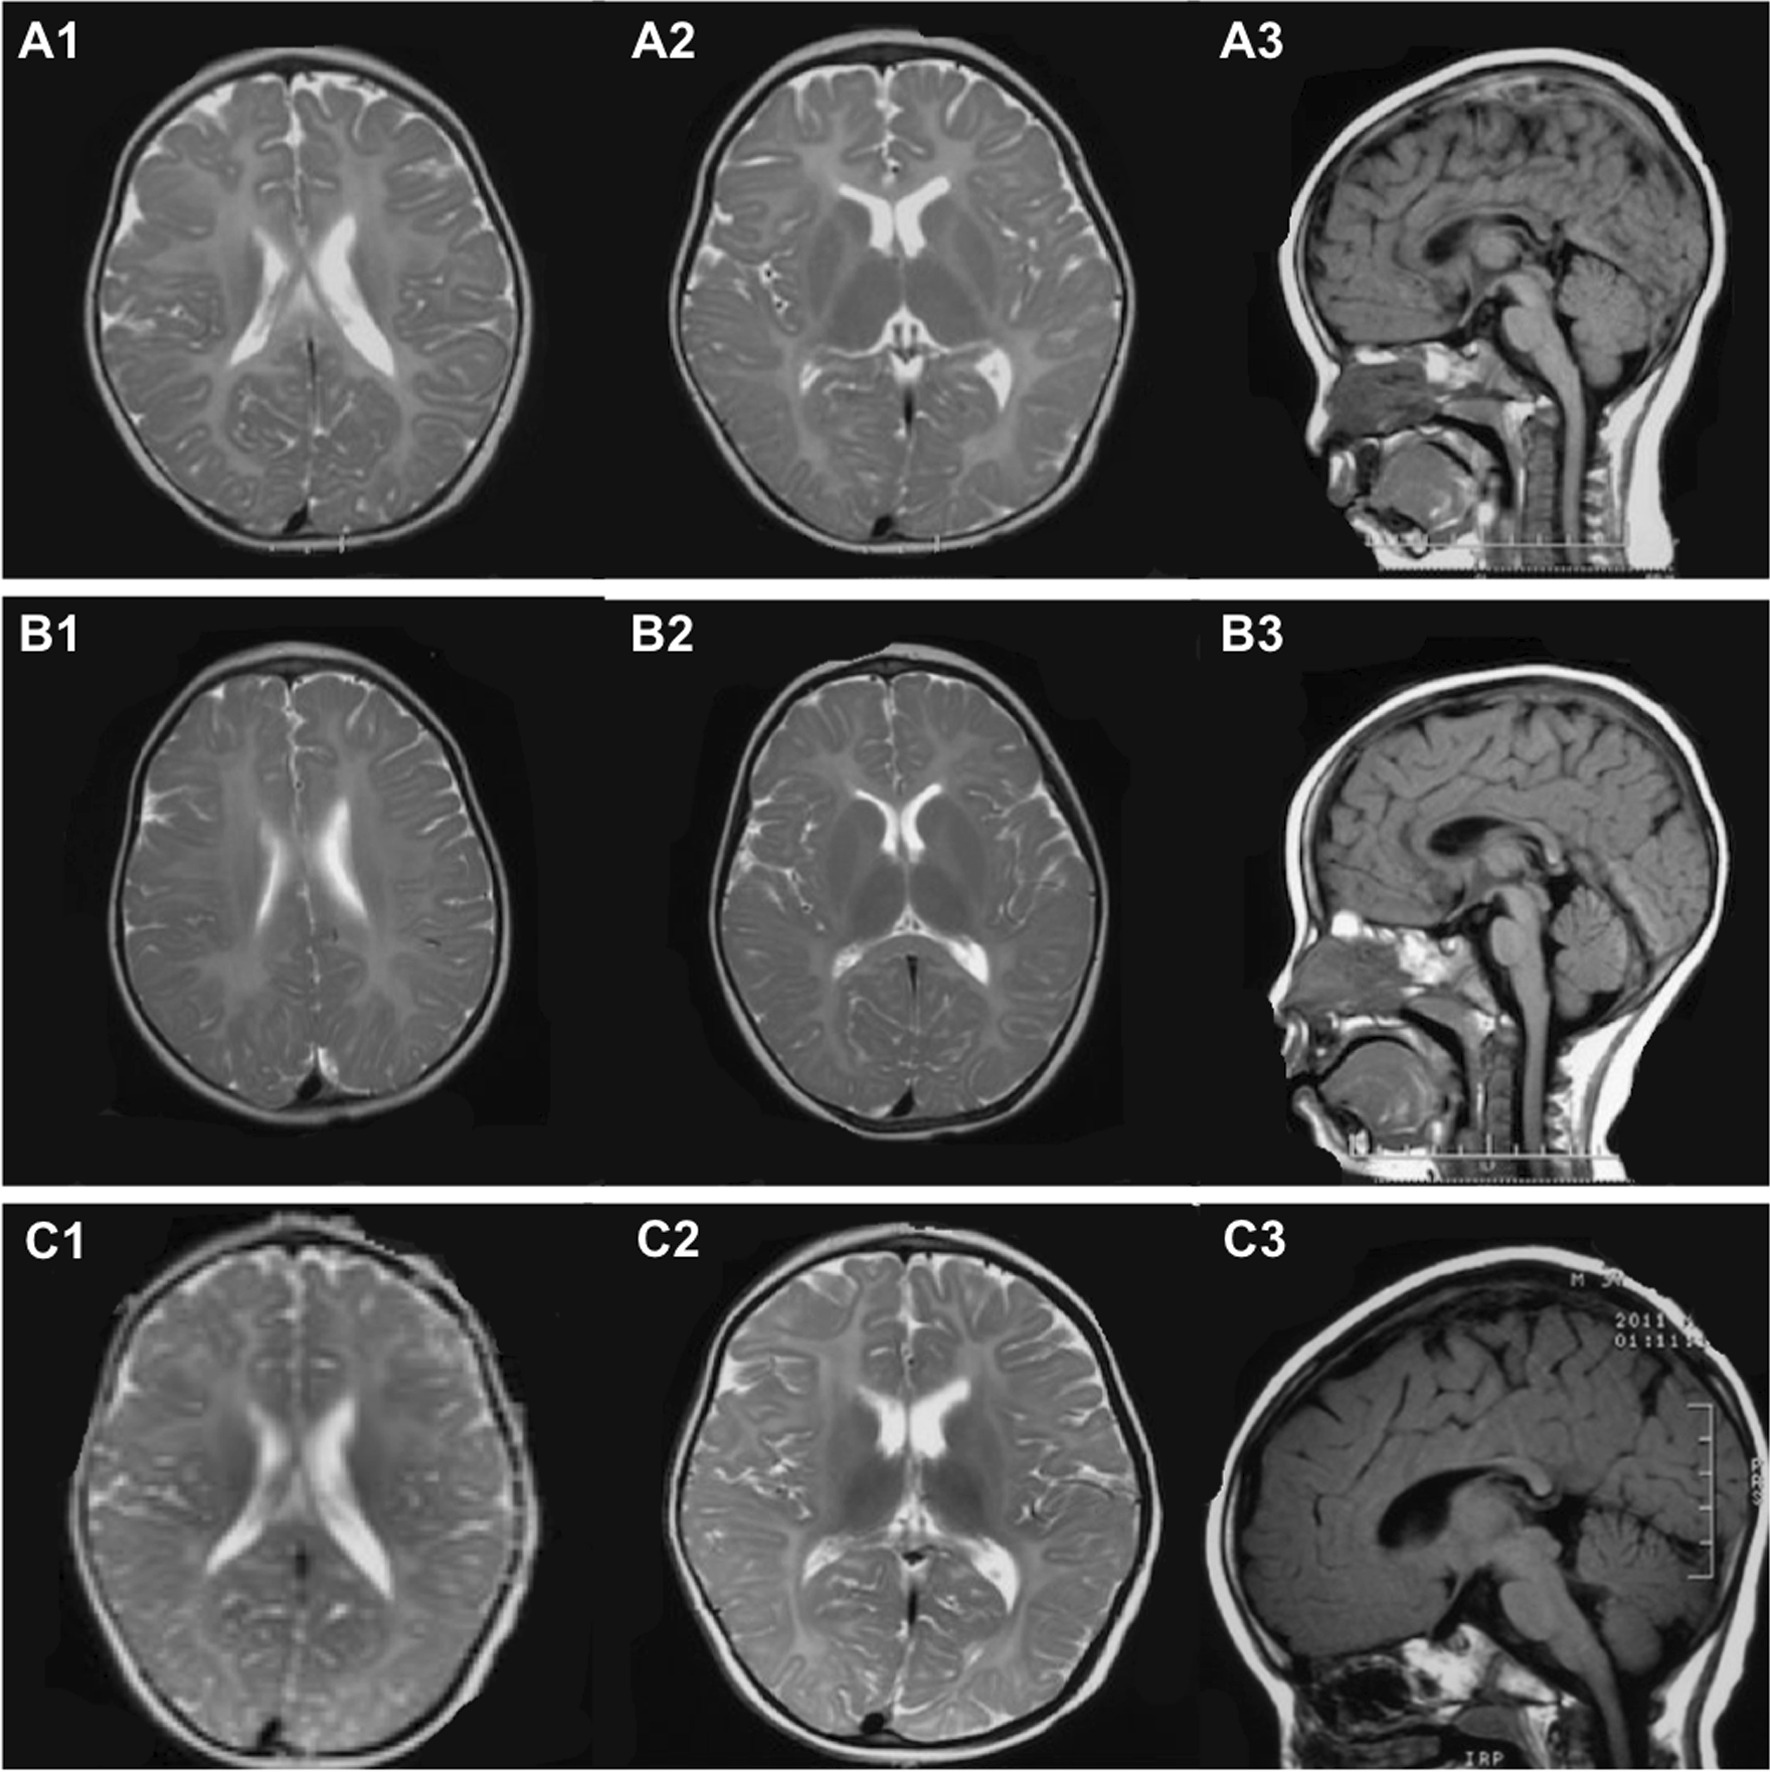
Brain MRI of patients with PMD. A1–A3, B1–B3, and C1–C3 represent the comparison of Pt28 brain images at 14 m, 30 m and 47 m, respectively. A1–A2, B1–B2, C1–C2 Diffuse hyperintensity in white matter presented in axial T2WI. A3, B3, C3 show atrophy of the corpus callosum on sagittal T1WI
